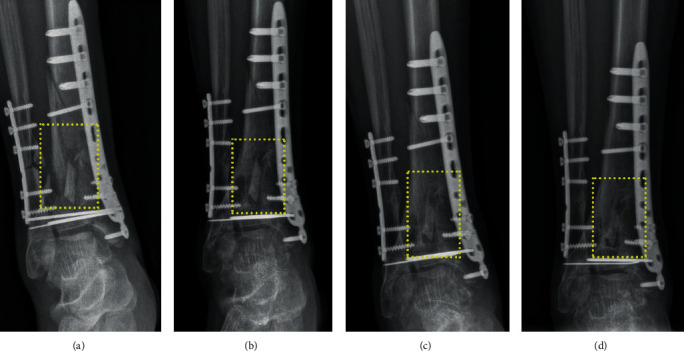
Plain radiographs of the right crus taken at the time when the patient was (a) admitted to the rehabilitation center, (b) before LIPUS therapy, (c) 1 week after the end of LIPUS therapy, and (d) 2 months after the end of LIPUS. The yellow dotted line box indicates the right distal tibial comminuted fracture region.
